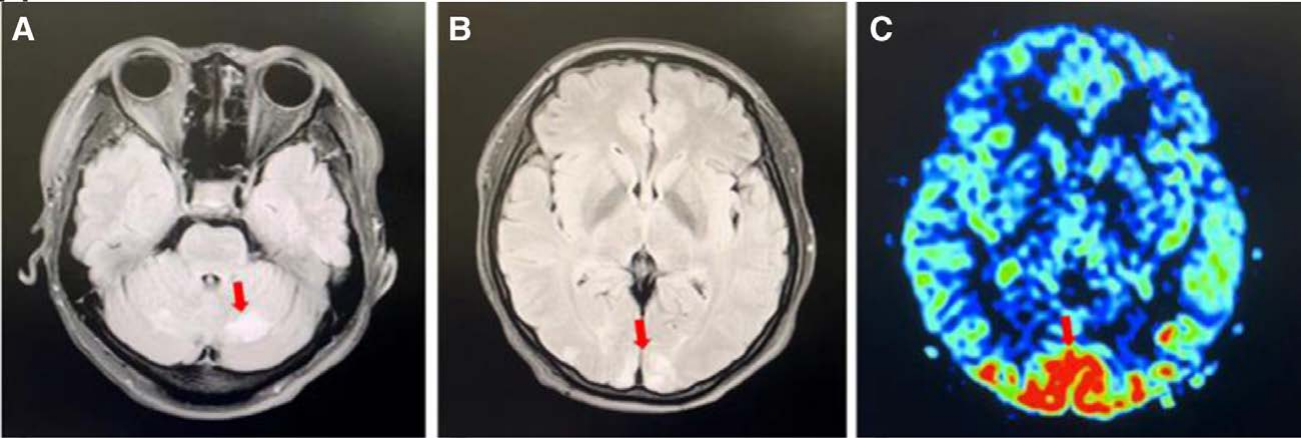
Brain MRI and cerebral blood perfusion images of the patient at the time of diagnosis of PRES. (A) Abnormal signal intensity in the posterior lobe of the cerebellum on MRI scan. (B) Abnormal signal intensity in the occipital lobe on MRI scan. (C) There is abnormal blood perfusion in the occipital lobe on functional MRI. MRI = magnetic resonance imaging, PRES = posterior reversible encephalopathy syndrome.
